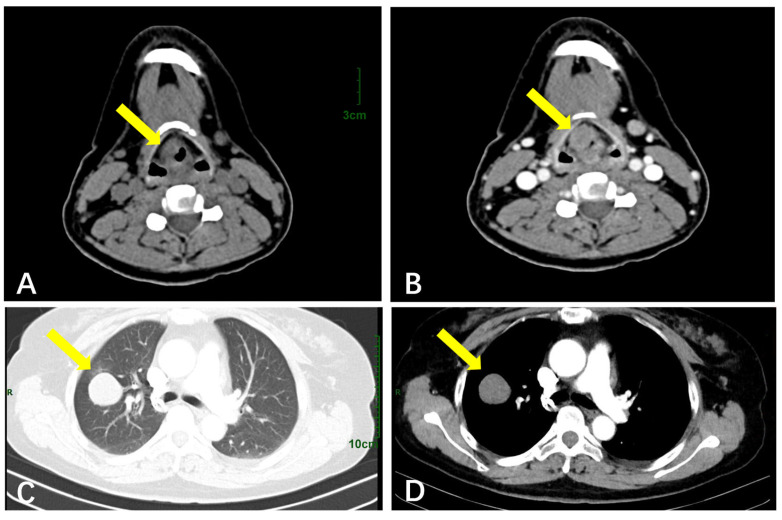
(A) Noncontrast CT scan showed a benign mass in the right larynx with well-defined soft tissue density opacity (yellow arrow); (B) CT with contrast showed enhancement within the mass; (C) noncontrast CT scan showed a malignant mass in the lung with clear borders (yellow arrow), and there was no significant difference in the image compared with the benign mass; (D) CT with contrast showed enhancement within the mass. (A,B) share a bar, (C,D) share a bar.
